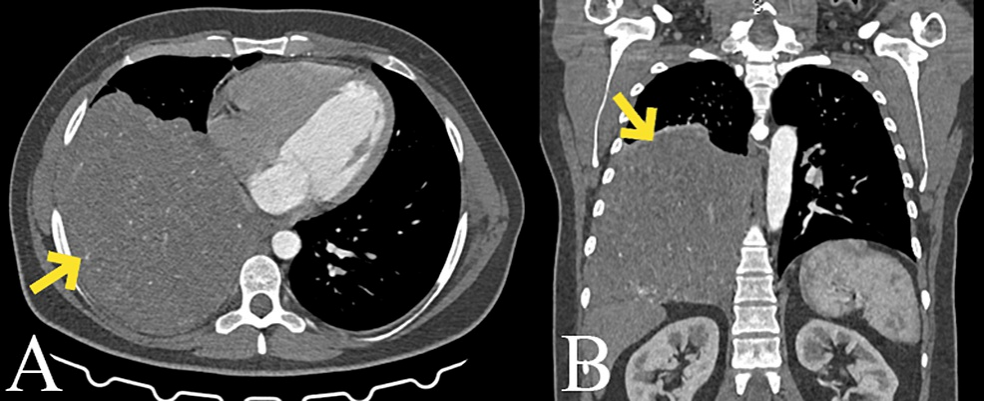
Arterial phase IV contrast enhanced axial acquisition 64 detector CT of the thorax showing a very large homogeneous mass in the right hemithorax. Non-enhancing areas corresponding to necrosis, myxoid degeneration, or hemorrhage within the tumor are not present. (A) Axial. (B) Coronal.
